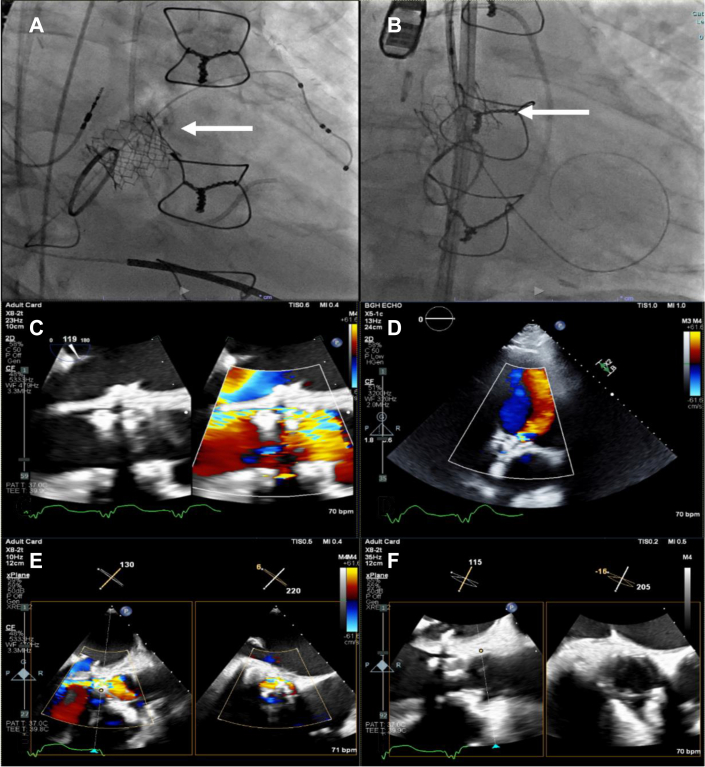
Imaging Demonstrating TAVR Valve Migration Migration of the TAVR valve into the LVOT (A); note the relative position of the TAVR valve with the previous SAVR (arrow). TAVR valve good position before migration (B). TEE at 119° showing severe paravalvular leak and migration of the TAVR valve down into the LVOT (C). TTE 4-chamber view showing severe paravalvular regurgitation in red (D). TEE at 130° X plane showing severe paravalvular regurgitation (E). TEE at 115° X plane showing the TAVR valve migrated into the LVOT (F). Note the short axis image at the aortic valve annulus level showing the SAVR valve leaflets without the TAVR valve present (indicating an incorrect position). LVOT = left ventricular outflow tract; SAVR = surgical aortic valve replacement; TAVR = transcatheter aortic valve replacement; TEE = transesophageal echocardiogram.
